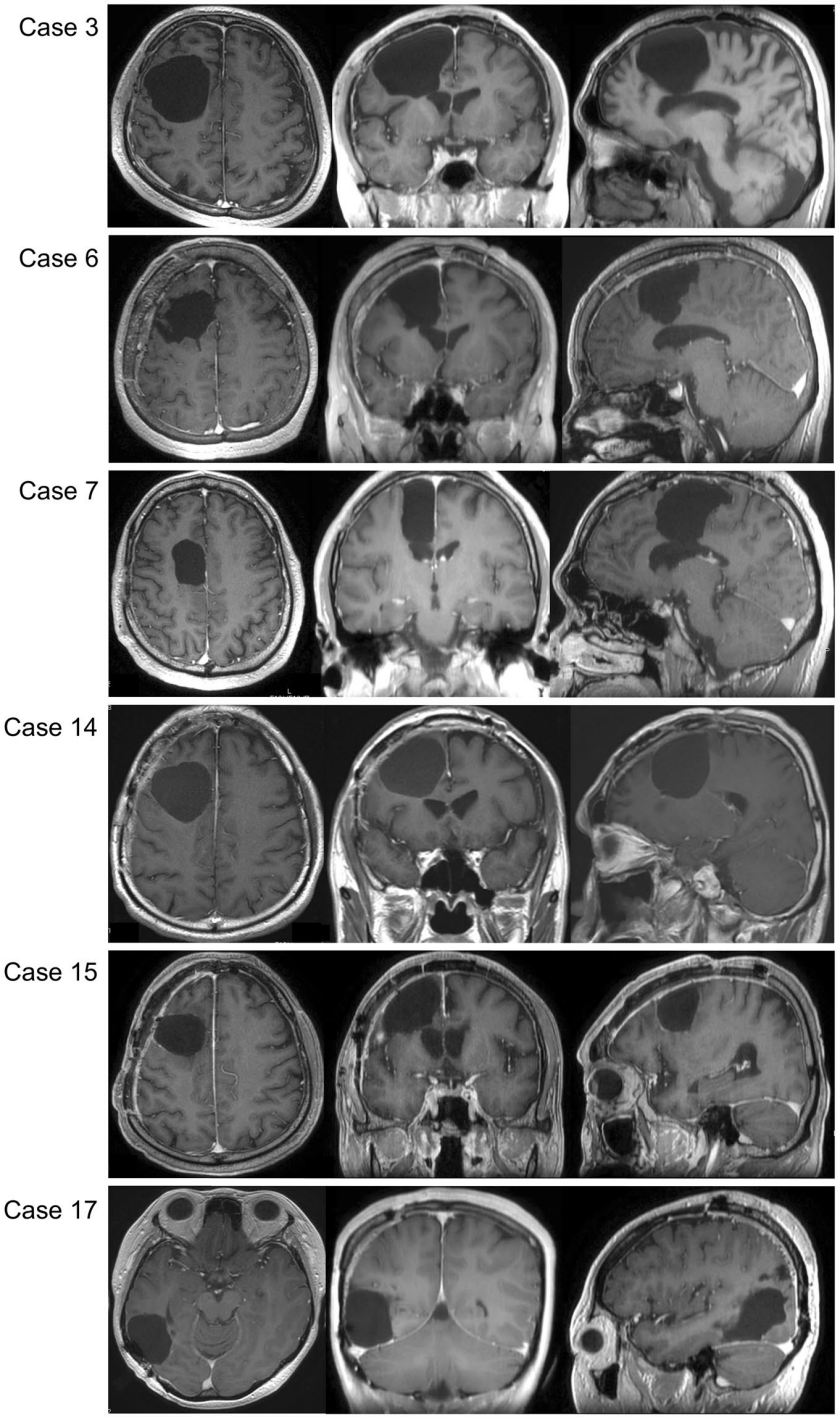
Postoperative MR images of patients whose visuospatial cognitive lasted ≥3 months. In the left, middle and right columns, T1 weighted MR images of axial, coronal, and sagittal slices, respectively, are shown for each patient. Resection cavities are in the middle frontal gyrus and/or superior frontal gyrus including the medial region in patients with frontal lesion.
